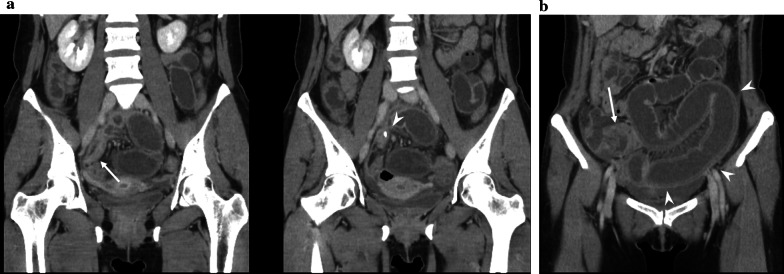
Abdominal contrast-enhanced computed tomography findings. a An appendix wall (white arrow) was thickened and there was a fecalith (white arrowhead) at the appendix root. b The cecum (white arrow) was edematous, and the small intestine in the pelvis (white arrowhead) was dilated
